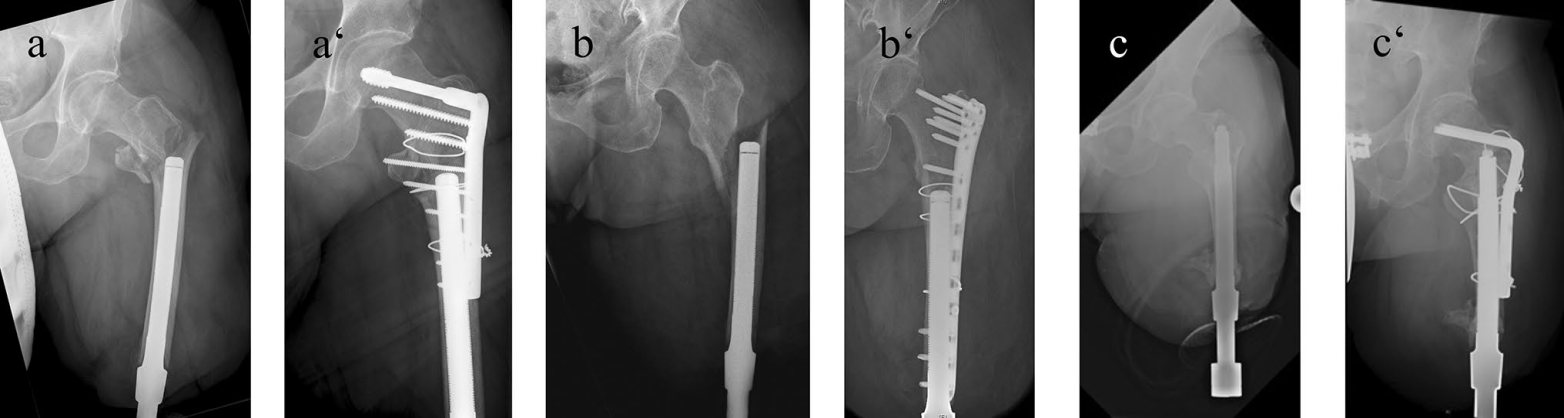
a–c X-rays with postoperative periprosthetic fractures before and after open reduction and internal fixation with plates and cerclage cable
